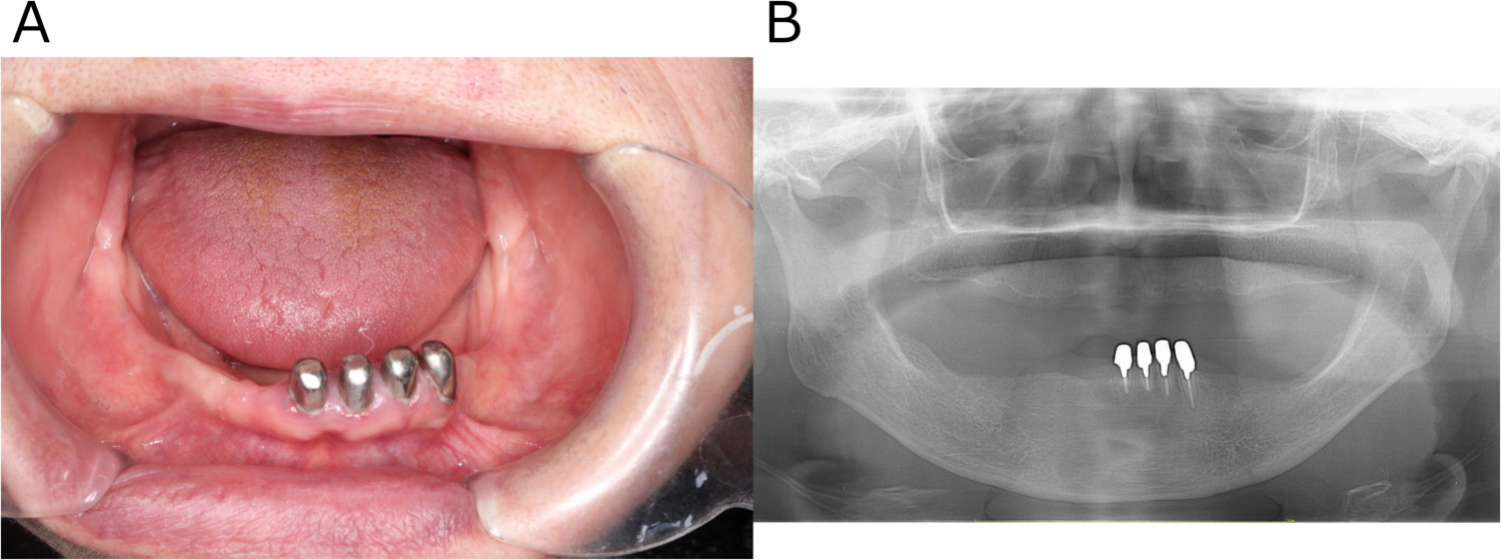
Clinical images obtained at the patient's initial visit to the psychosomatic dentistry clinic. (A) Intraoral photograph showing no structural or organic abnormalities that could explain the reported pain. (B) Panoramic radiograph likewise revealing no structural or organic abnormalities responsible for the pain.
